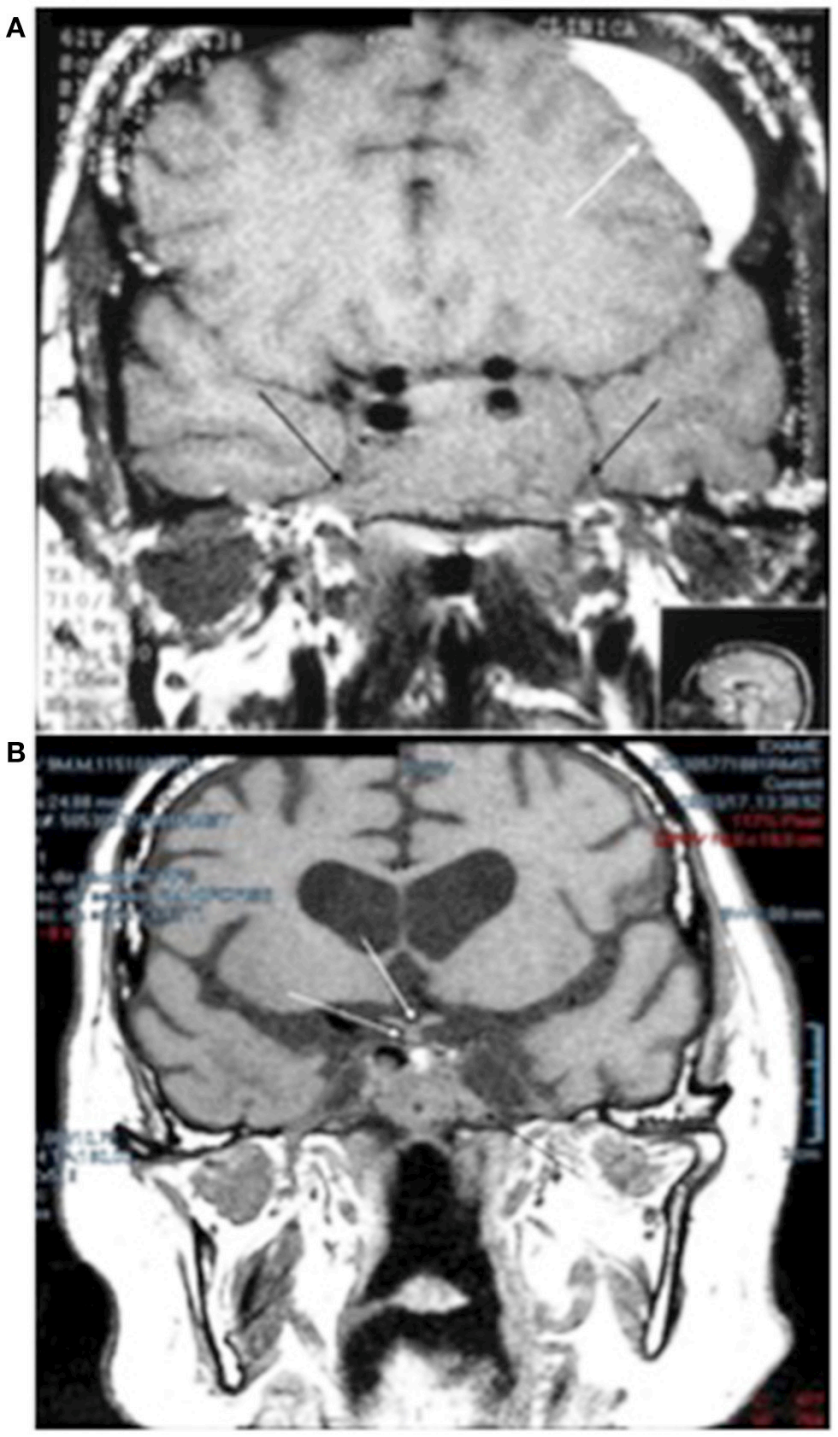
(A) Magnetic resonance imaging of the sella turcica, on T1, without contrast, prior to starting treatment with cabergoline, which shows a pituitary adenoma with total invasion of the sphenoid sinus and cavernous sinuses on both sides (black arrows), measuring 4.0 × 2.5 x 2.5 cm. The image also shows a subacute subdural hematoma in the left frontal lobe of the brain (white arrow). (B) After 17 years of treatment with cabergoline, a reduction in the size of the pituitary adenoma can be seen with visualization of the optic chiasm and pituitary stalk (white arrows); tumor remnants invading the sphenoid sinus (black arrow) are visible.
